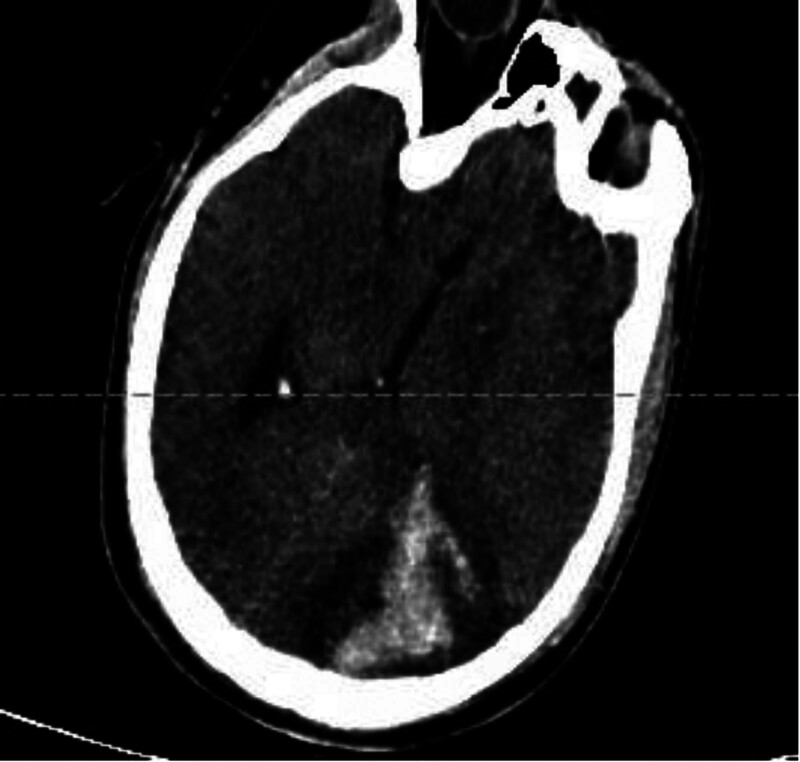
Cranial computed tomography.
